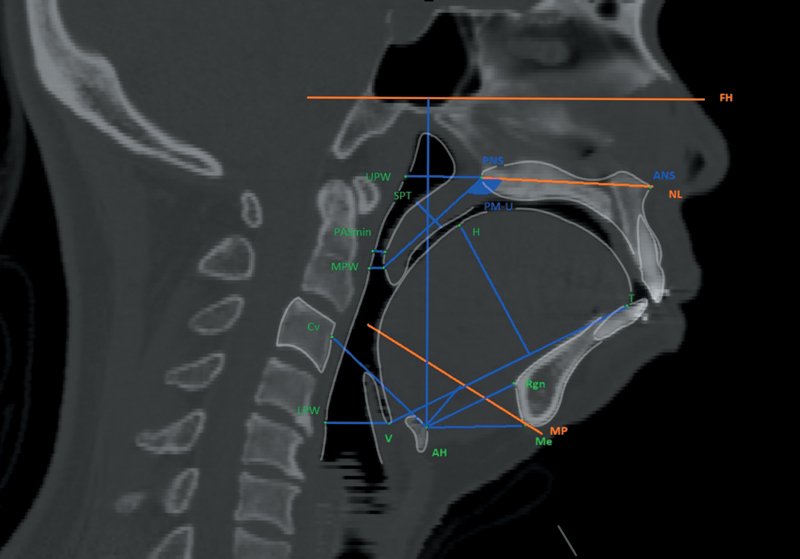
Lateral cephalometric images from spiral CT scans in the mid-sagittal plane and corresponding measurements.
